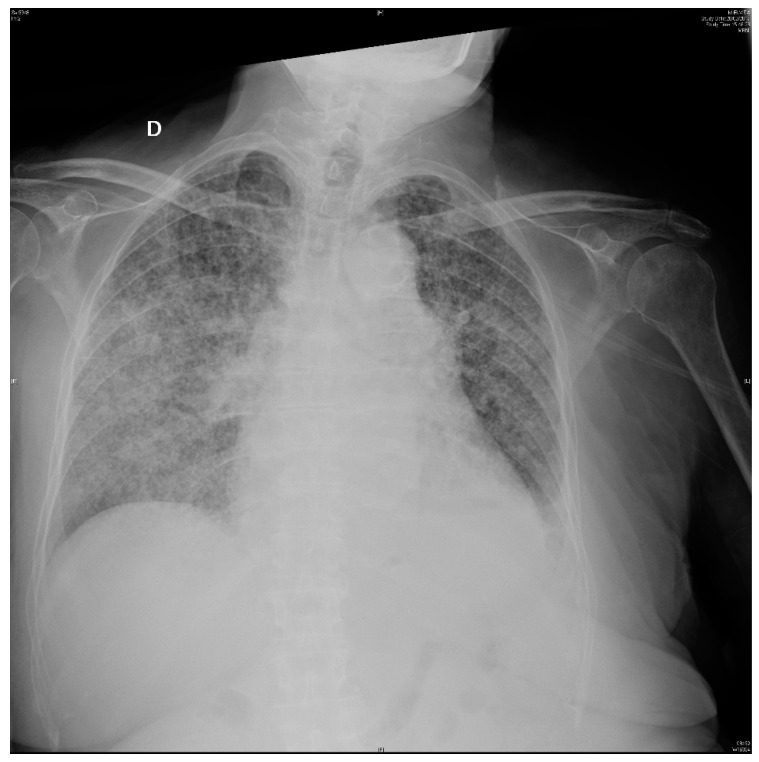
Chest X-ray on 16th day: Extensive bilateral reticulo-nodular infiltrates.
